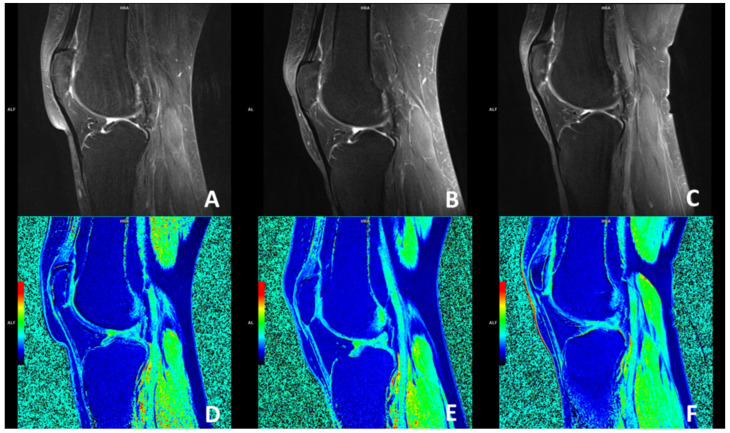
Sagittal MRI magnetic resonance imaging (MRI) slices through the center of the knee accessing the patellofemoral joint osteoarthritic changes using fat-suppressed proton-density-weighted turbo spin-echo method at baseline (A), 3 months (B), and 6 months (C) follow-ups with corresponding delayed gadolinium-enhanced MRI of the cartilage (dGEMRIC) images (D–F). No changes were seen when dGEMRIC indices were calculated.
